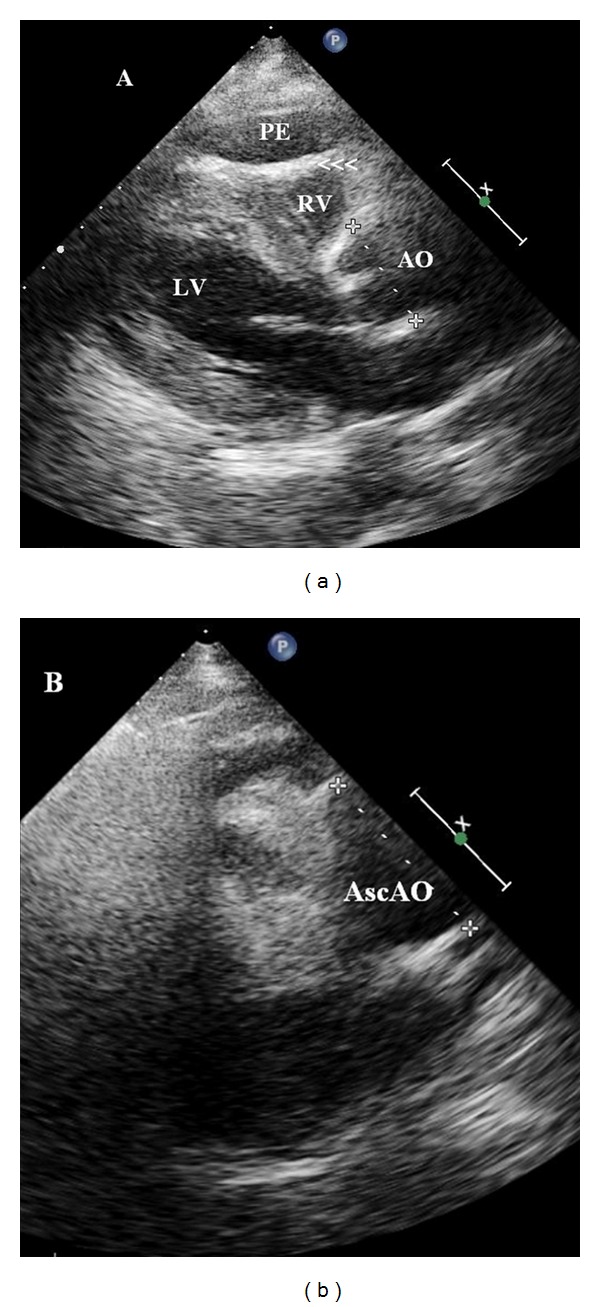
Transthoracic echocardiogram in parasternal long axis view showing moderate pericardial effusion with diastolic collapse of right ventricle (A, arrowheads) in a patient with ascending aortic dissection. Note markedly dilated ascending aorta (B, 5.9 cm). LV: left ventricle; RV: right ventricle; AO: aorta; PE: pericardial effusion; AscAo: ascending aorta.
